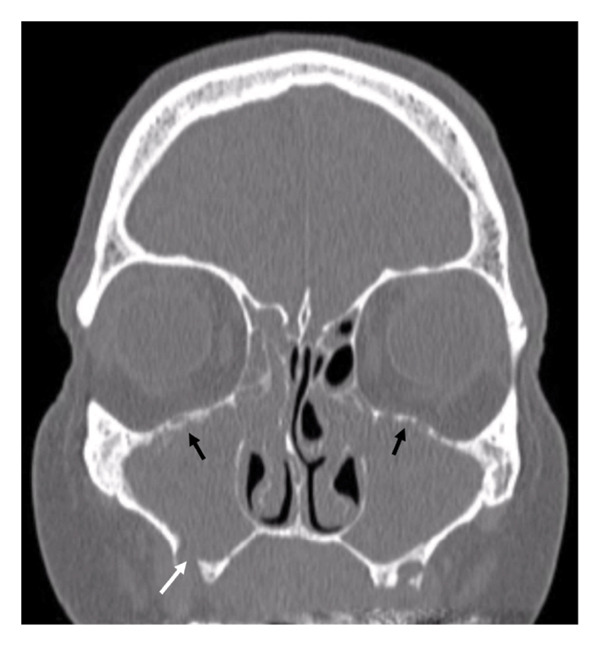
Computed tomography of the paranasal sinuses prior to surgery. The white arrow indicates the tooth extraction site with a bone defect. Black arrows indicate areas of osteitis in the superior walls of the maxillary sinuses.
